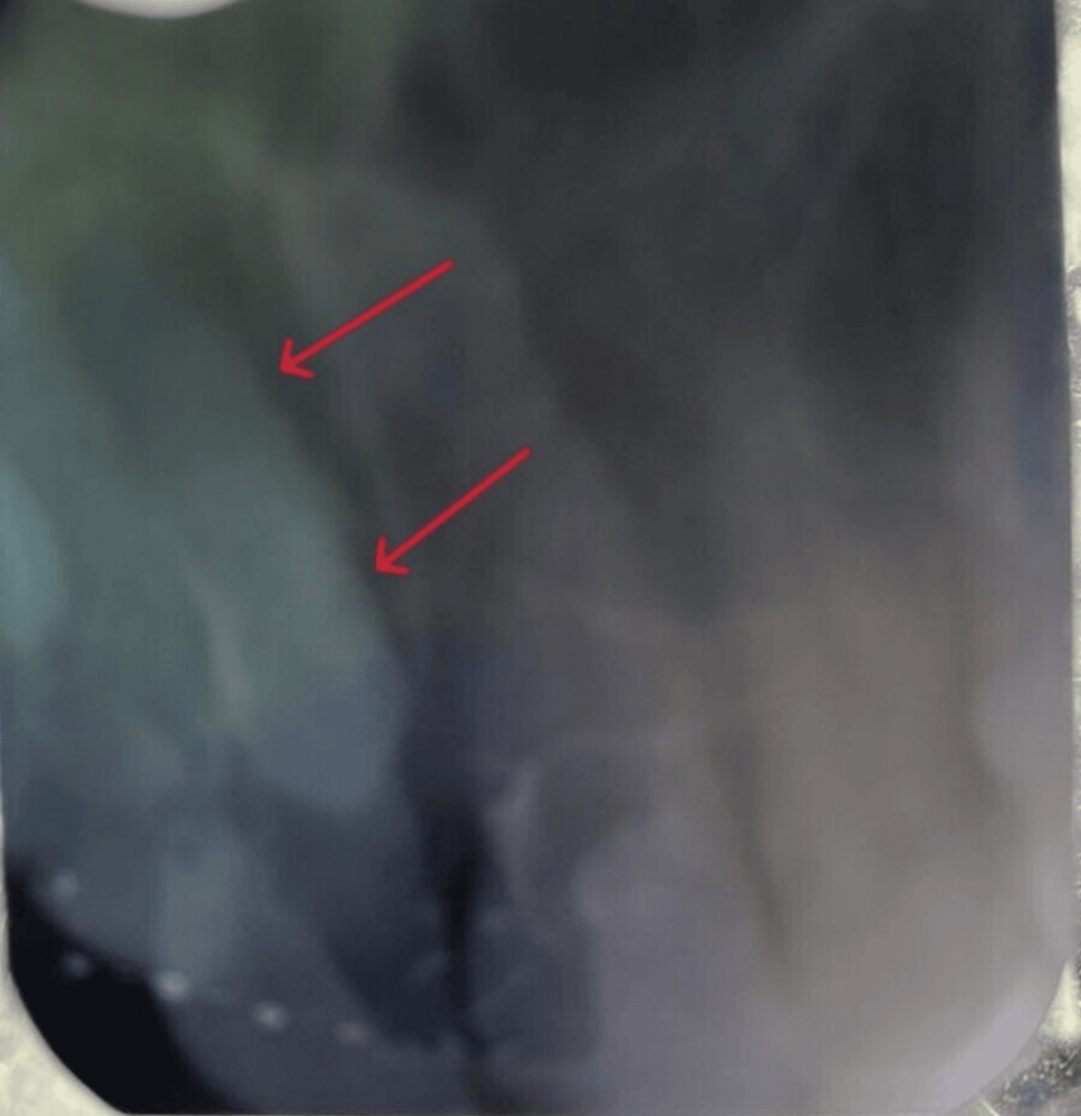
Intraoral radiograph of the maxillary anterior region showing the mesiodens (red arrows)
